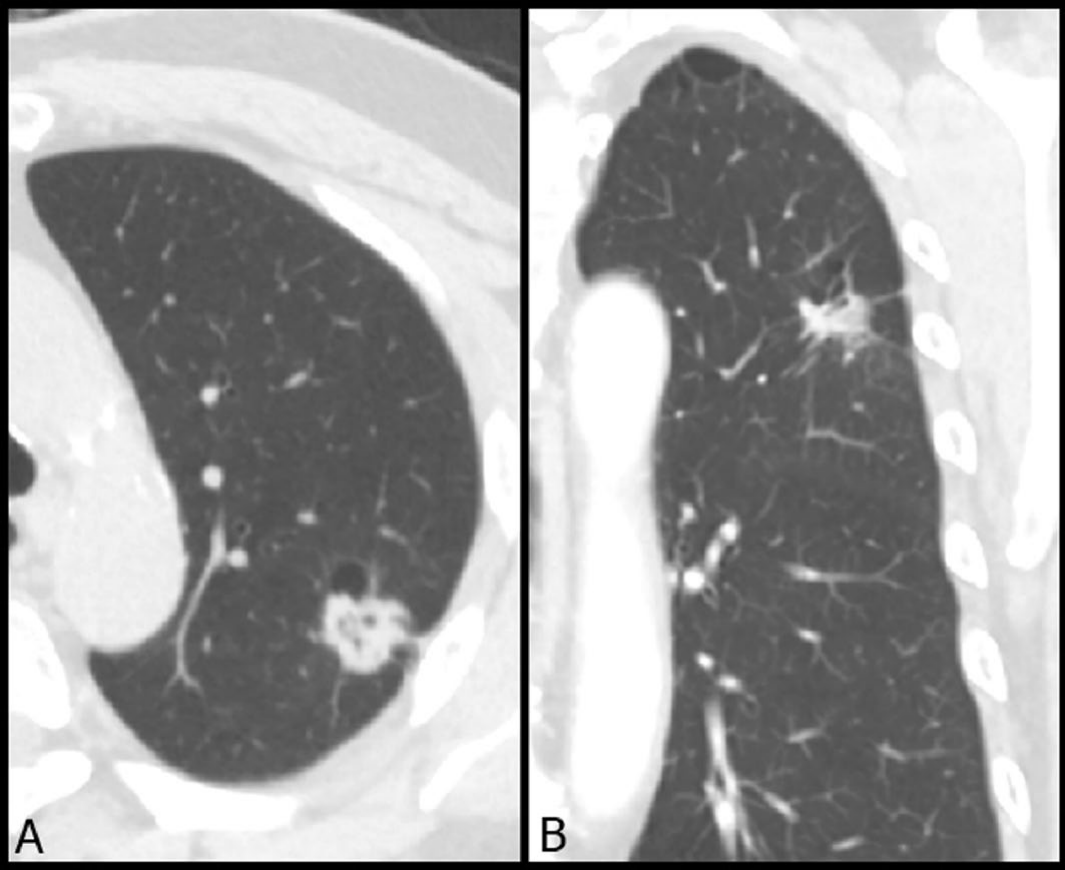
A typical example case of subsolid nodule with abnormal cystic-like airspace in LUL. A 65-year-old man had a 2.1 cm part-solid nodule with spiculated border in LUL. The (A) axial and (B) coronal CT images showed an abnormally dilated cystic-like airspace inside the lesion. The patient underwent video-thoracoscopic lobectomy of LUL. Further pathologic report demonstrated invasive pulmonary adenocarcinoma in LUL, Stage T1cN0M0. *LUL* left upper lobe.
